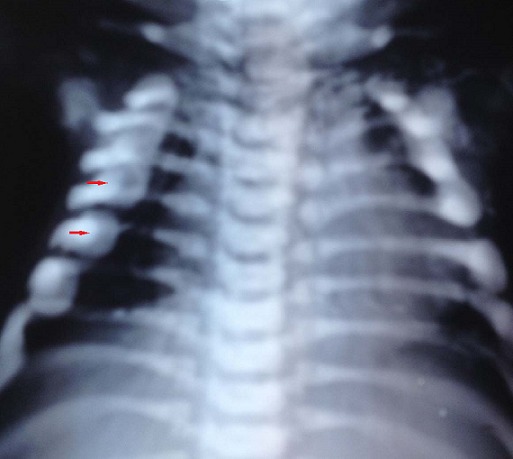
Chest radiograph. Note rachitic rosary
